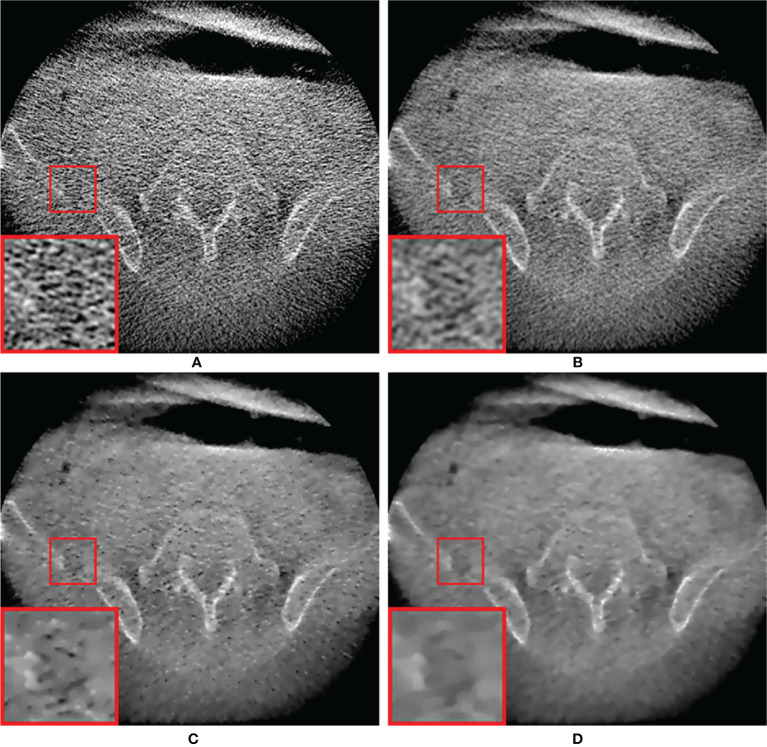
Comparison between the same views of CBCT images generated by applying the analytical reconstruction algorithm based on (A) PDB, (B) RDB, (C) RDB followed by NLTV, and (D) RDB followed by MI-NLTV using a patient data with the pelvic bone metastatic lesion. These CBCT images are displayed at the window settings of (width = 2,400, level = 400) HU.
